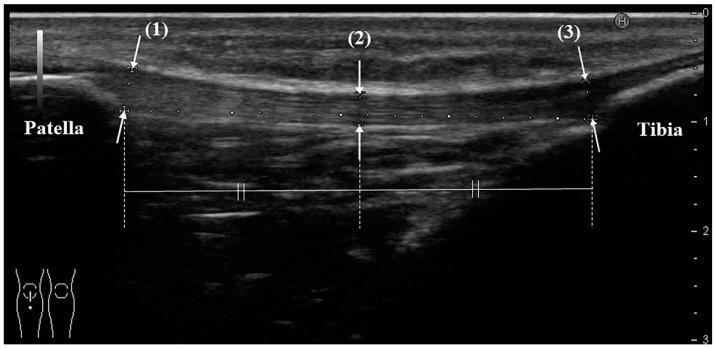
Measurement method of patellar tendon thickness with ultrasonography (long-axis image). Thickness is defined as the distance from the superficial to the deep layers of the tendon, measure at: (1) the proximal part: the point where the deep layer of the tendon is attached to the lower end of the patella; (2) the midpoint between the proximal and distal parts; and (3) the distal part: the point where the deep layer of the tendon is attached to the tibial tubercle. All schemes followed the same formatting.
